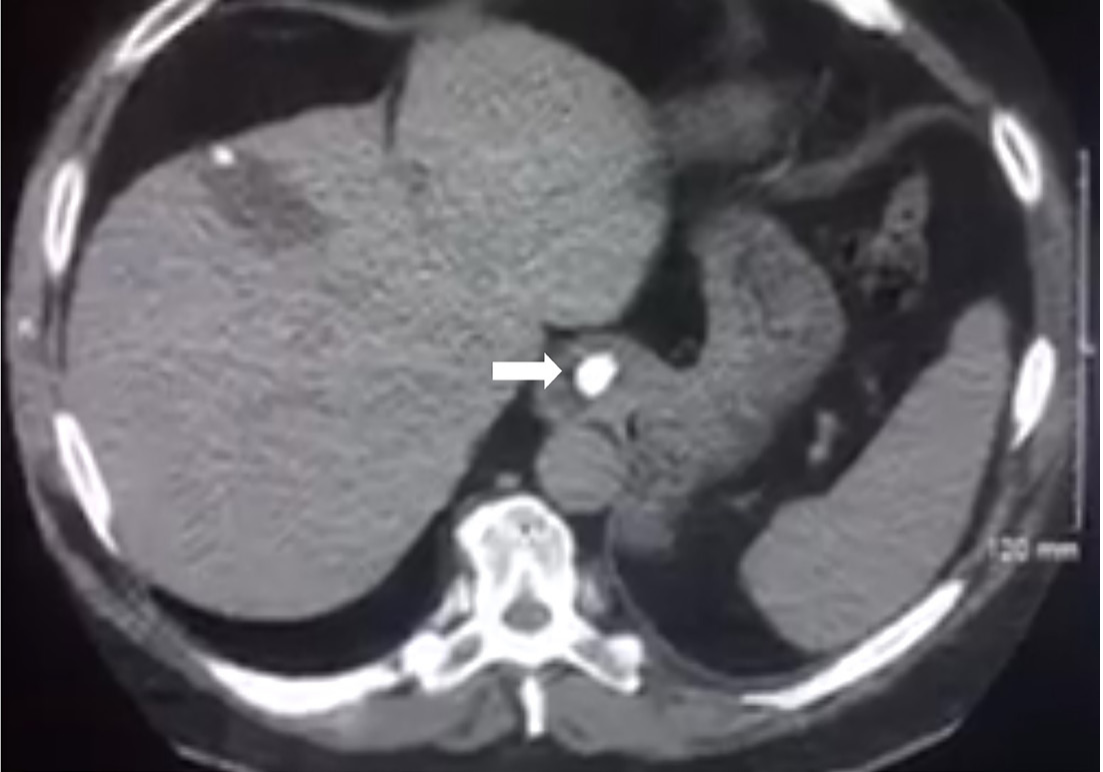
Image of this patient’s computed tomography with the foreign body located near the gastroesophageal junction (arrow).
